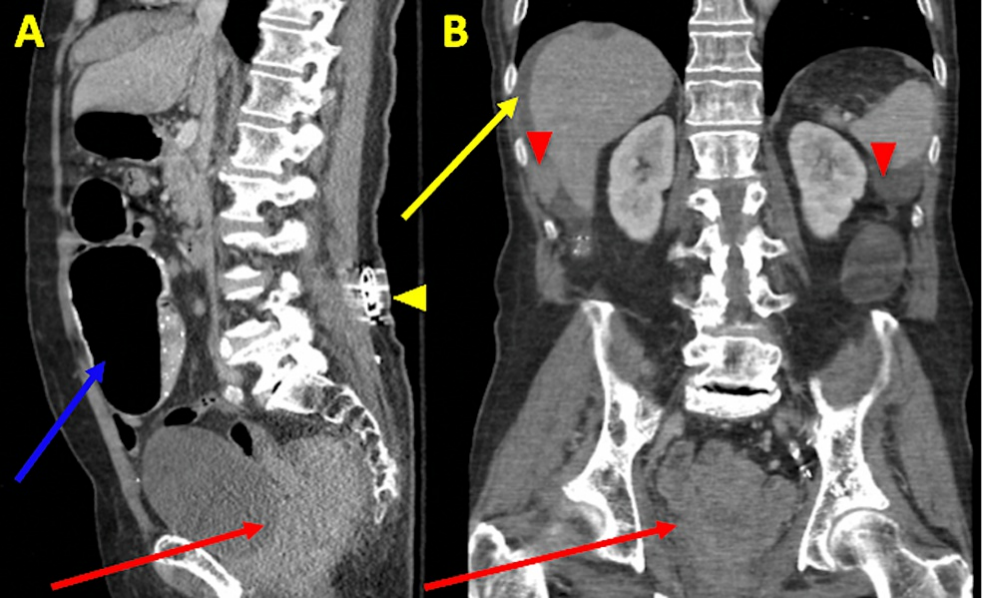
Contrast CT imaging of the abdomen and pelvis showing a rectal mass and evidence of metastasis Sagittal (A) and coronal (B) images of the abdomen and pelvis showing wall thickening of the rectum with a large mass lesion obliterating the surrounding tissue planes along the rectosigmoid colon and encasing the rectum measuring approximately 5.6 cm x 7.6 cm (red arrows). The colon is dilated suggesting distal obstruction (blue arrow). There is a moderate amount of perihepatic fluid (yellow arrow) and multiple nodules throughout the abdomen concerning metastatic disease (red arrow tips). There is a sacral nerve stimulator (yellow arrow tip).
